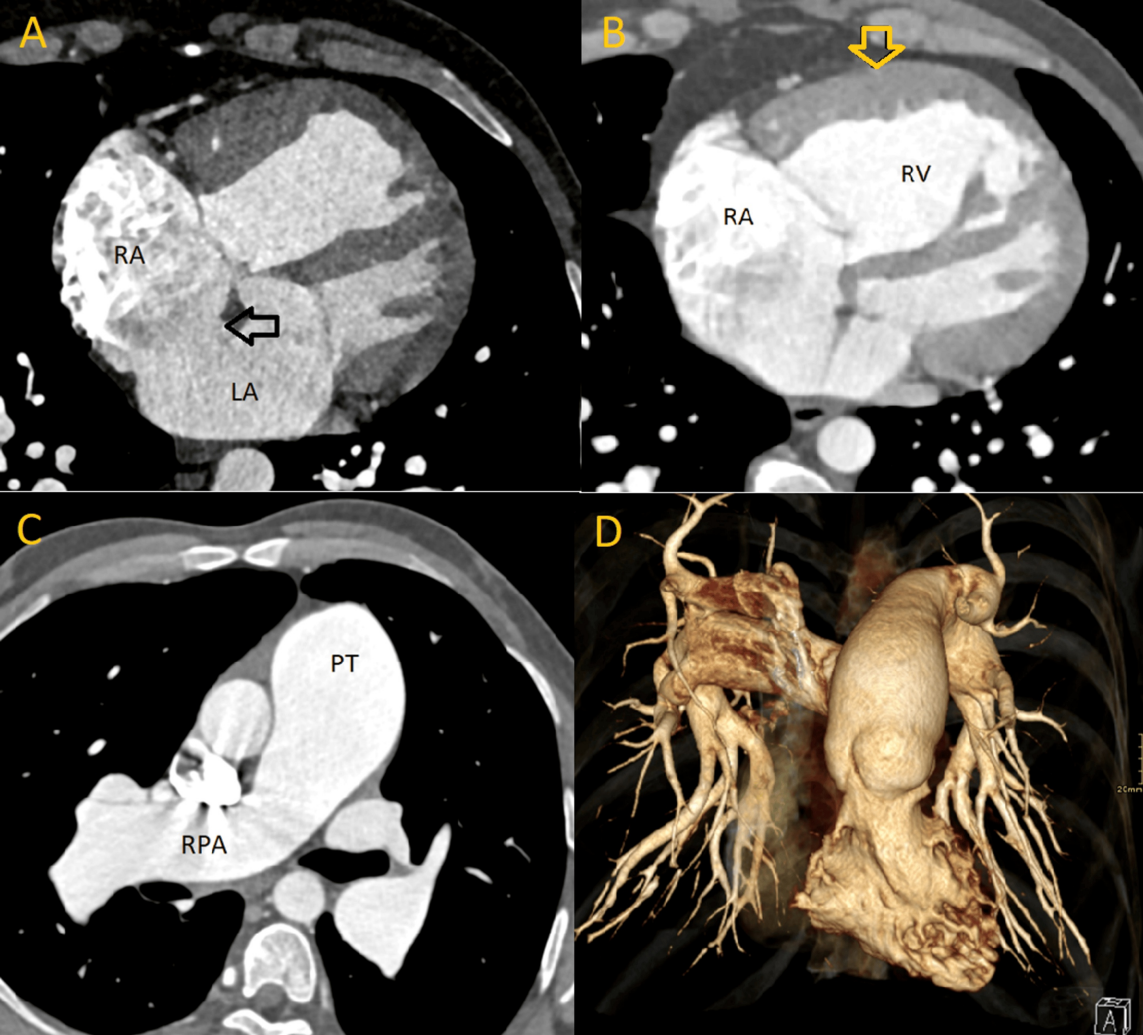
CT images of the heart and pulmonary arteries. A) long-axis view of the heart with ASD (black arrow); B) right heart chambers with thickened myocardium (yellow arrow); C) pulmonary truncus and right pulmonary artery; D) 3D reconstruction of the pulmonary arteries RA: right atrium; LA: left atrium; RV: right ventricle; PT: pulmonary truncus; RPA: right pulmonary artery
